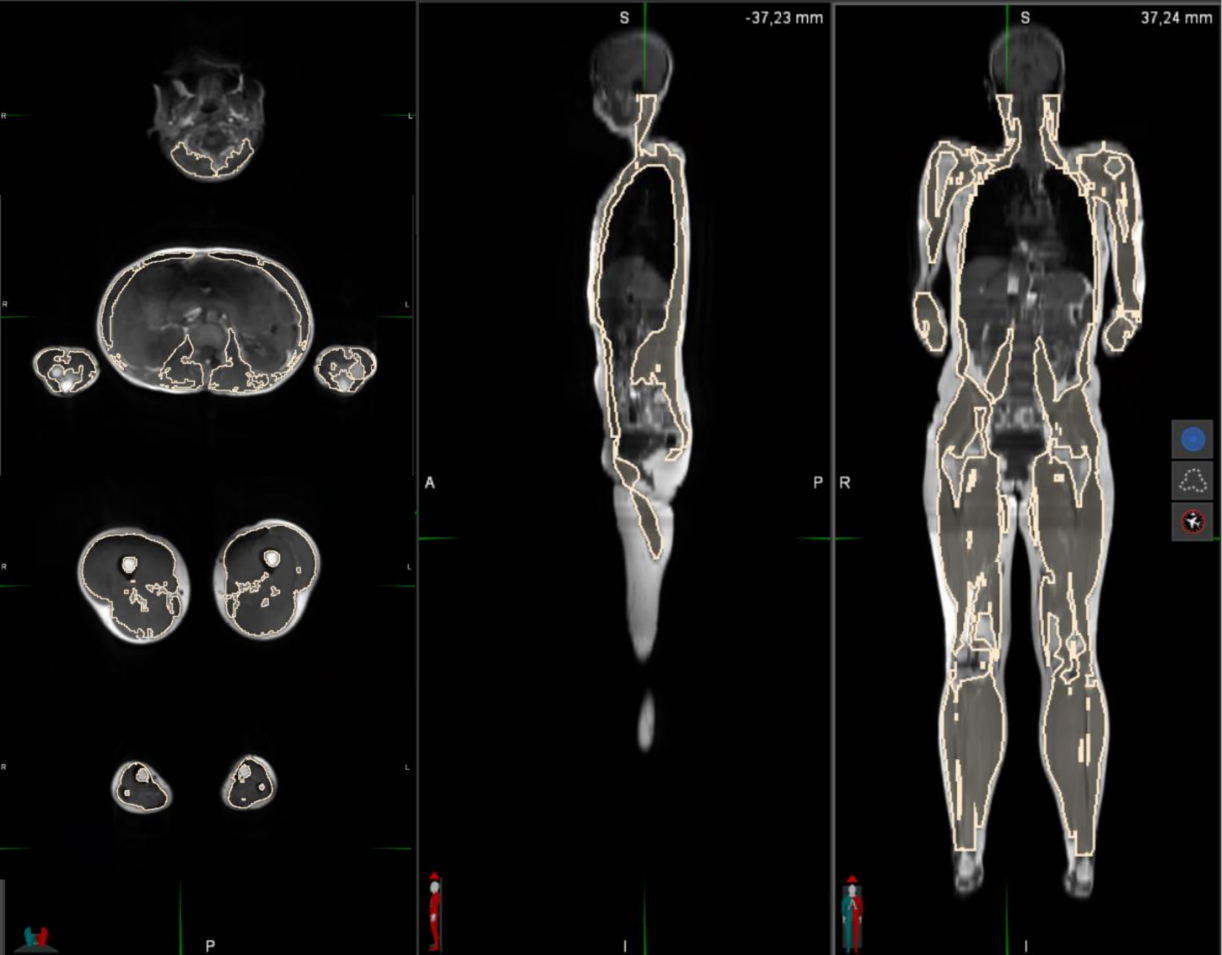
Contoured muscles on MRI. Female participant, 28 years old, height: 1.65 m, weight: 58 kg, muscle mass: 21.2 kg.
